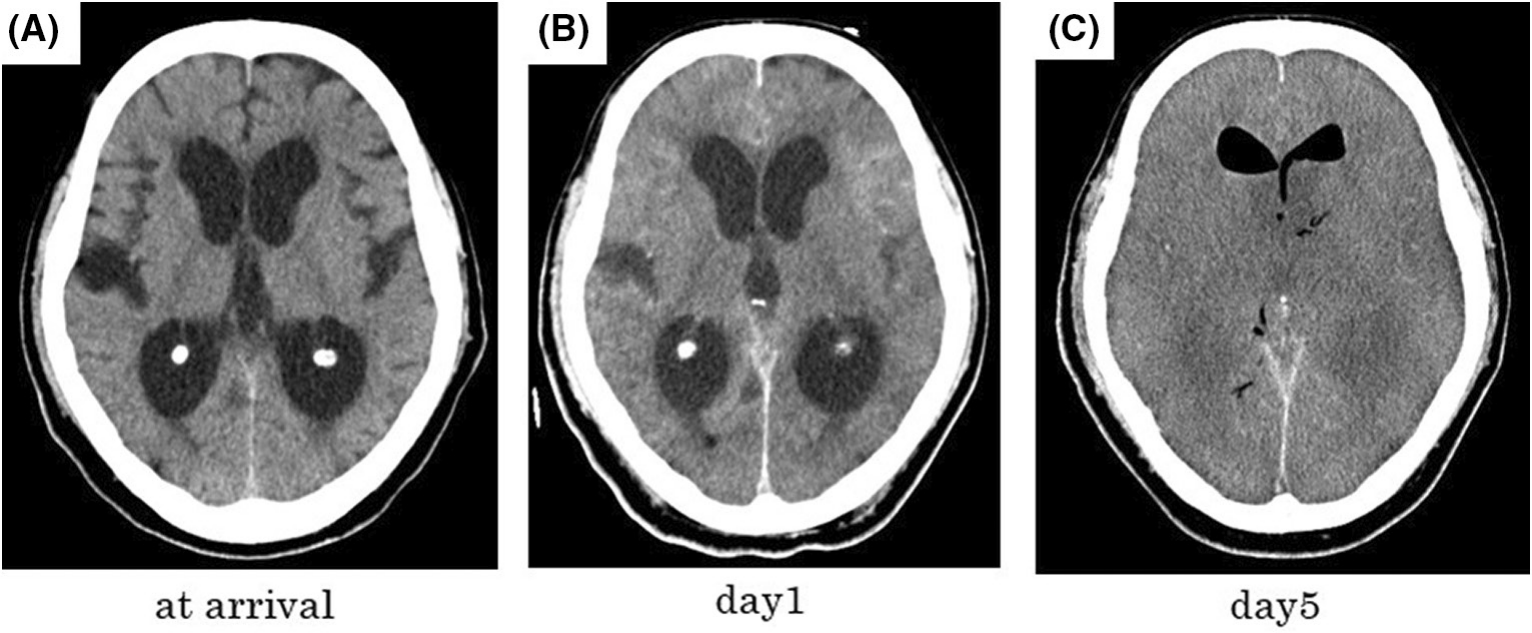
(A‐C) Head computed tomography scans in Case 3 showing diffuse sulcal effacement over time and air replacement in the ventricles. (A) at arrival (B) on day1 of hospitalization (C) on day5
